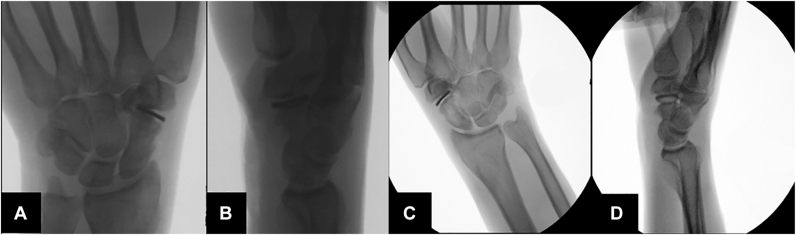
Perioperative fluoroscopic x-rays. A, B First implantation. C, D Second revision.
